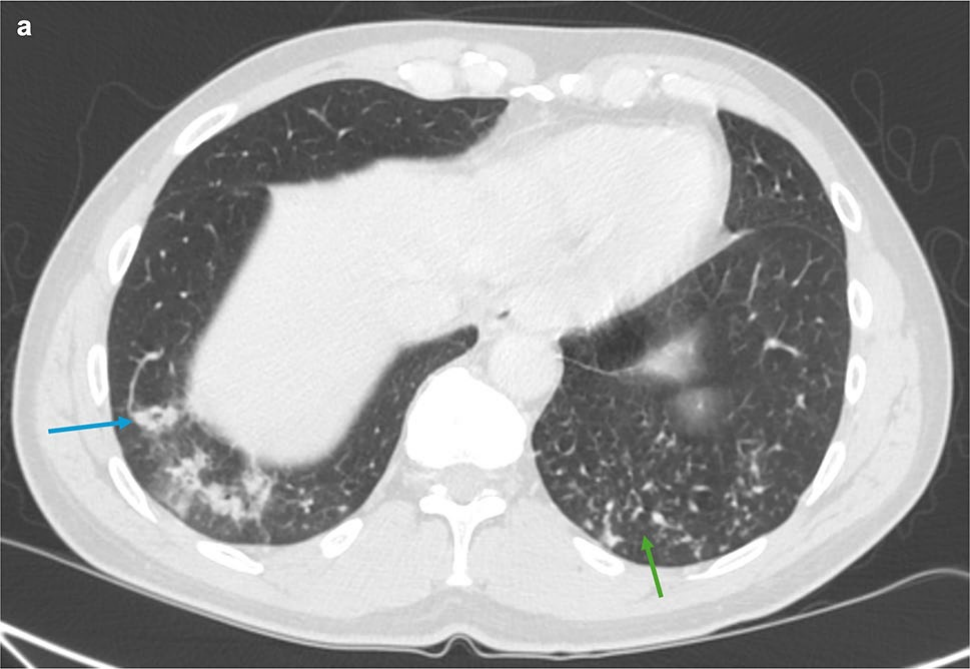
HRCT of the chest (a) showing cryptogenic organizing pneumonia, which typically demonstrates the reverse halo sign (blue arrow), nodular tree-in-bud lesions (green arrow), patchy ground-glass opacities, and inflammation of bronchioles and alveoli. These are all suggestive of an organizing pneumonia pattern
